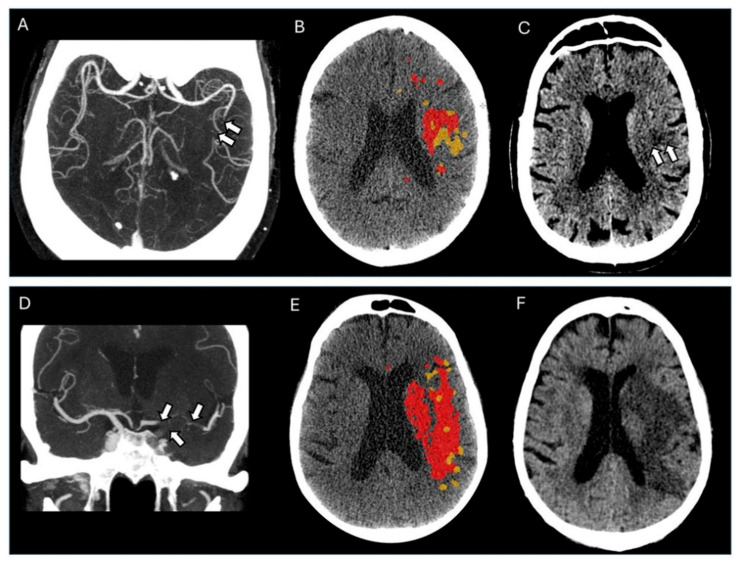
(A–C) A patient with a distal thrombus. (A) An axial CTA view shows an occlusion in the M2 segment of the left middle cerebral artery (arrows), resulting in a CBS of 9. The thrombus length was 12.5 mm. (B) CTP demonstrates a small core volume (red) and a small hypoperfused volume (yellow). (C) An NCCT at 1-day follow-up shows a small final infarct volume (arrows). (D–F) A patient with a proximally located thrombus. (D) A CTA coronal view showing a thrombus in the left ICA-t segment, extending to the A1 and proximal and distal M1 segments (arrows). The CBS was 3, and the thrombus length was 23.6 mm. (E) CTP shows a large core volume (red) and hypoperfused volume (yellow). (F) A 48-h follow-up NCCT demonstrates a large final infarct volume.
